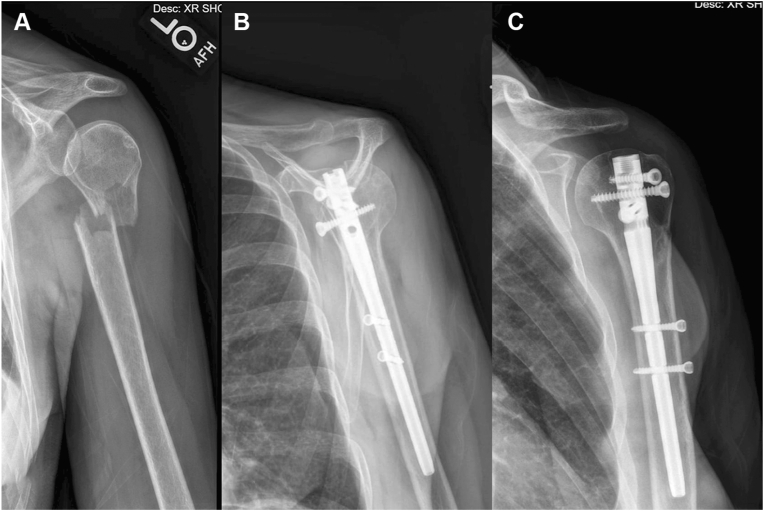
X-ray showing (A) a fracture of the surgical neck of the humerus, (B) scapular Y and (C) AP views showing intramedullary nail fixation. *AP*, anteroposterior
